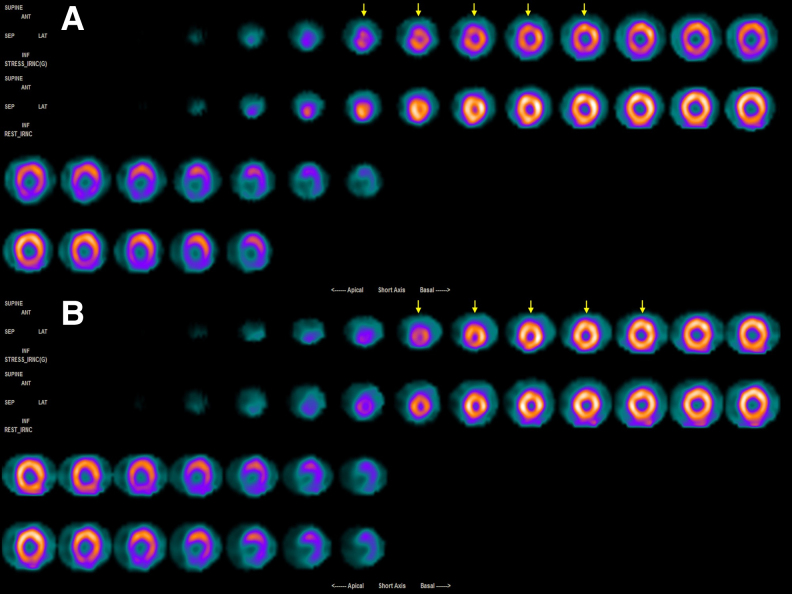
Myocardial perfusion scintigraphy images showing anterior ischaemia before the procedure (*A*) and normal perfusion following the procedure (*B*). Arrows on both panels point to the anterior left ventricular wall, where exercise-induced ischaemia can be seen on preoperative (*A*) but not on the postoperative (*B*) images.
